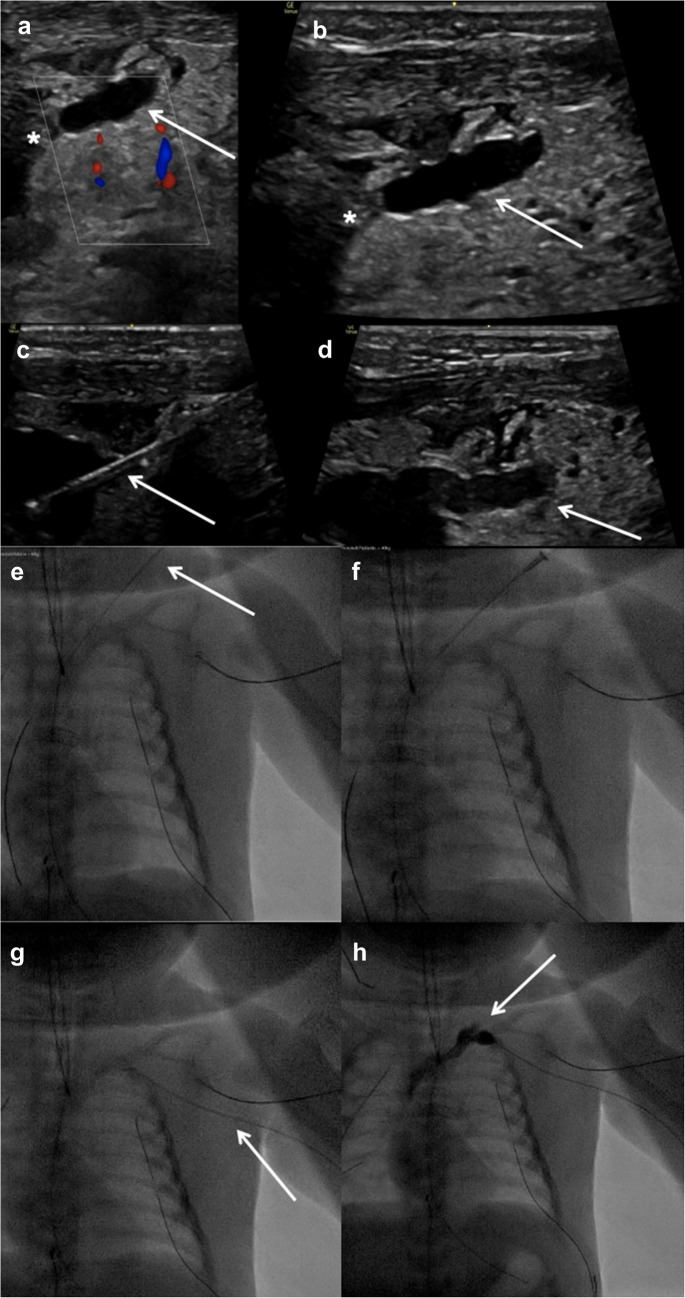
A 3-kg female neonate with high-output chylothorax. a-d Longitudinal ultrasound images demonstrate a thrombus (*asterisk*) occluding the outflow of the dilated thoracic duct (*arrow*) at the thoracic duct–venous junction with (a) and without (b) color Doppler. Under ultrasound guidance, the thoracic duct was punctured via the left neck with a 22-G peripheral venous catheter and a 0.014-in. floppy guidewire (*arrow* in c) was advanced through the needle and the occluding thrombus. The thoracic duct was cleared of thrombus at its venous junction (*arrow* in d). e–f Cropped anteroposterior fluoroscopic chest radiographs demonstrating the procedure. After removal of the peripheral venous catheter from the guidewire (*arrow* in e), the access tract was sequentially dilated with a 20-G cannula followed by a 14-G peripheral vein catheter using the Seldinger technique. Mechanical thrombus mobilization is demonstrated on the anteroposterior fluoroscopic chest radiograph (f). A catheter (*arrow* in g) was introduced via the left brachial vein, with its tip positioned at the thoracic duct–venous junction demonstrated on the fluoroscopic chest radiograph. Correct catheter placement is confirmed after contrast administration, with opacification of the left brachiocephalic vein and superior vena cava on fluoroscopic chest radiograph (h). Minimal opacification of the thoracic duct (*arrow* in h) is noted, consistent with backflow from the venous junction into the duct
